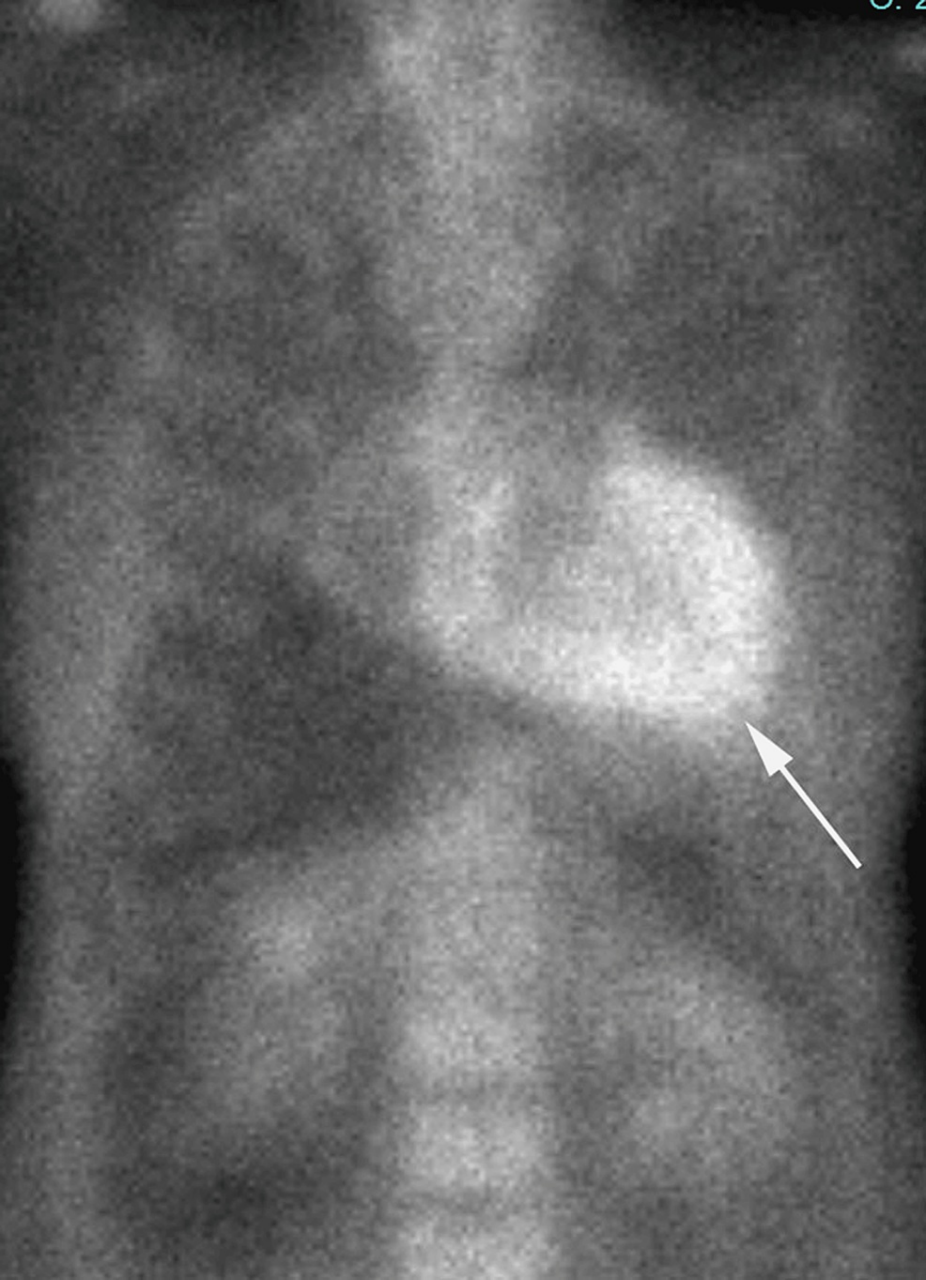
Anterior planar images of Tc-99m PYP scintigraphy Anterior planar images of Tc-99m PYP scintigraphy with intense myocardial uptake of PYP (visual score of 3) and high background activity in a patient with known chronic kidney disease. Tc-99m PYP: technetium-99m pyrophosphate
